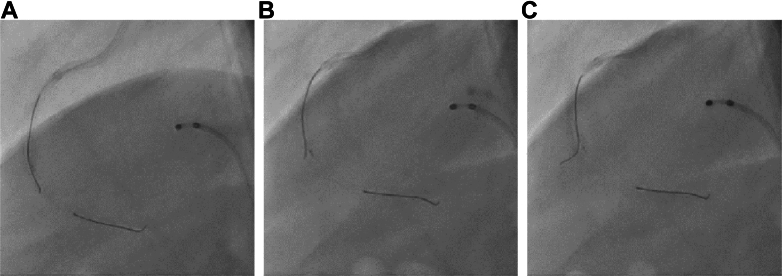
Wiring After the COA View Technique The tip of the guidewire is pointed left at a position slightly beyond the bifurcation (A). It is gradually withdrawn, and when the tip enters the acute marginal branch, its behavior changes (B). The guidewire is advanced in this position (C). COA view, calculation of the optimal angiographic view using intravascular ultrasound. COA view = calculation of the optimal angiographic view using intravascular ultrasound.
